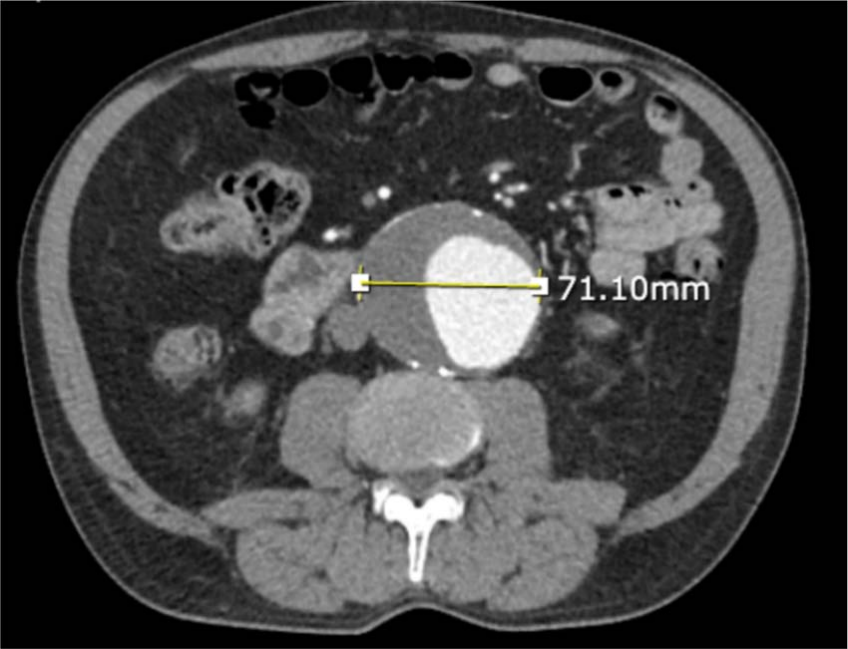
CT angiogram of abdominal aorta and iliofemoral artery axial view.
